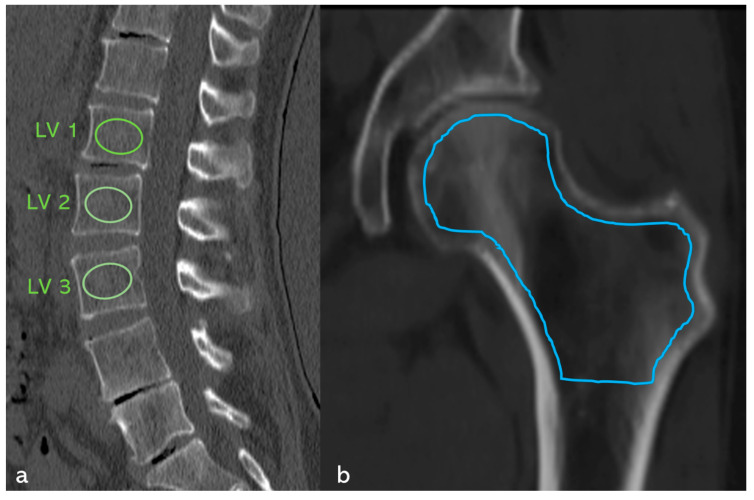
Measurement of cancellous density in HU. (a) Sagittal reformatted CT slice of the lumbar spine with positioning of the ellipsoidal ROI (green) in the mid-vertebral layer of LV1, LV2, and LV3. (b) Coronary reformatted CT slice of the left hip with positioning of the irregular ROI (blue) in the proximal femur.
